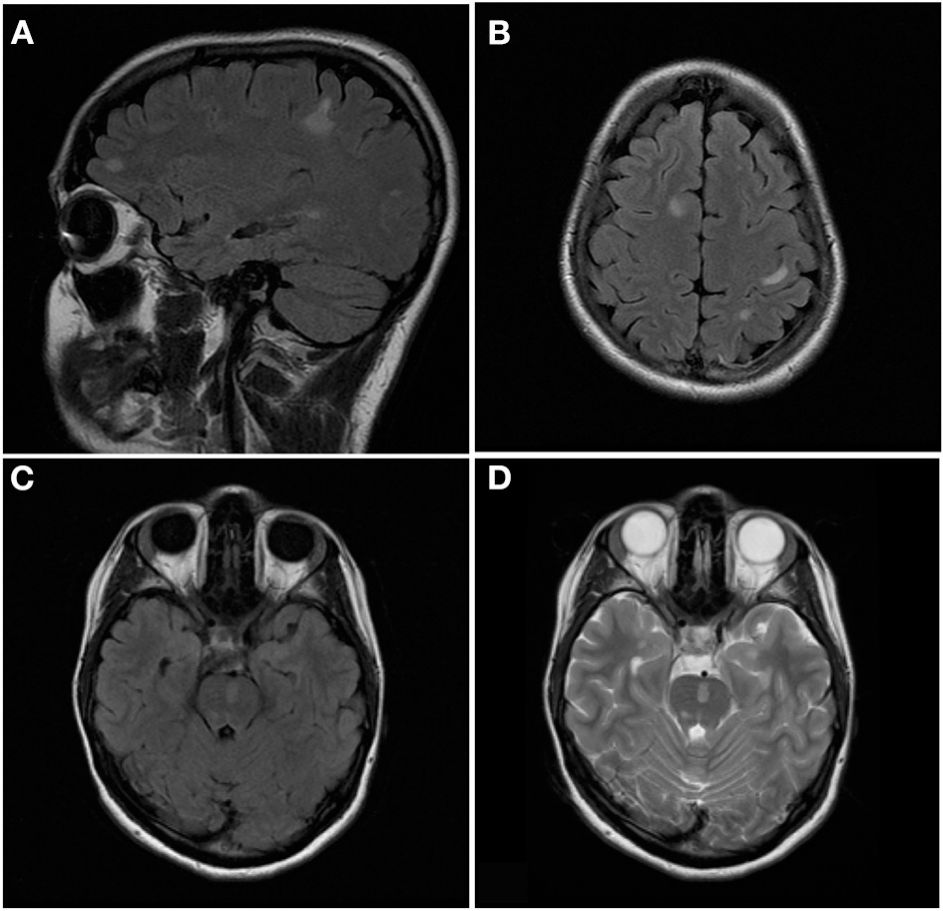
Sagittal FLAIR (A), axial FLAIR (B,C), and axial T2 (D) images of the brain from the same patient at the time of disease presentation. (A,B) Multiple FLAIR hyperintense lesions involve the juxtacortical and subcortical white matter of both cerebral hemispheres. (C,D) Additional T2/FLAIR hyperintense lesions involves the juxtacortical white matter of the left temporal lobe and pons.
